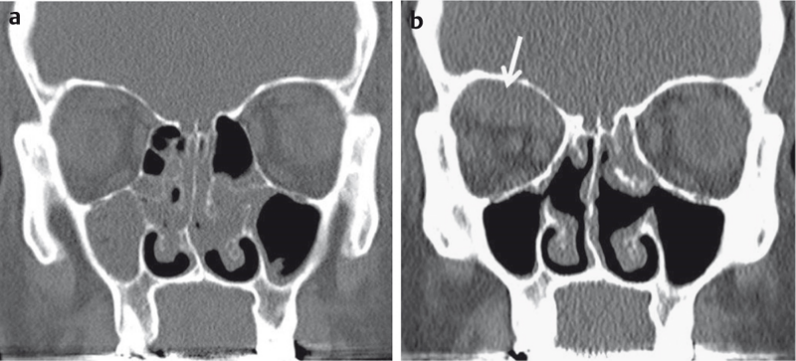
63-year-old diabetic patient with exacerbation of chronic rhinosinusitis. a) CT of the paranasal sinuses, coronal reconstructed image in bone window-level setting. Complete opacification of the right maxillary sinus and obstructed left ostiomeatal complex. b) Follow-up CT two weeks later after conservative therapy. New onset of headache and diplopia. Coronal reconstructed image in bone window-level setting demonstrates a smoothly marginated subperiostal lesion. Abscess formation was confirmed by surgery.
